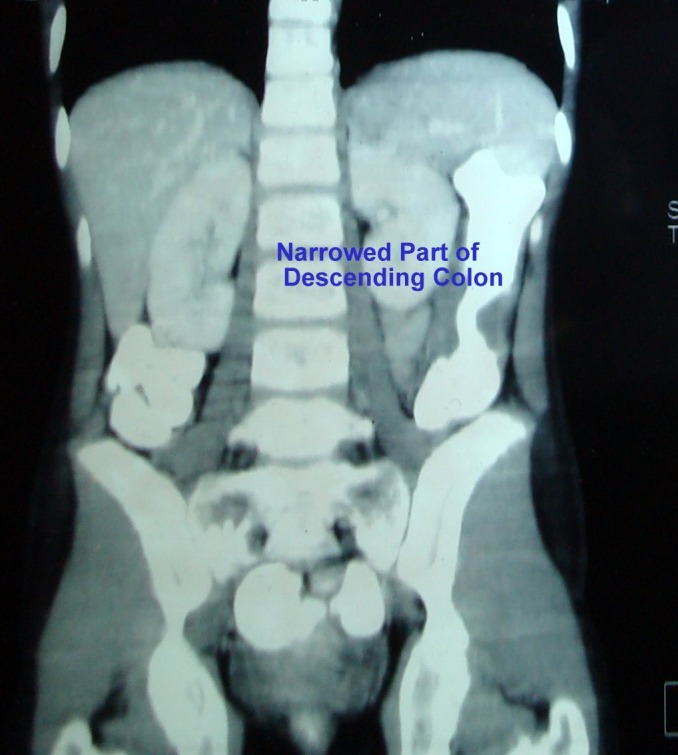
Figure 3: CT scan showing narrowing of descending colon.
